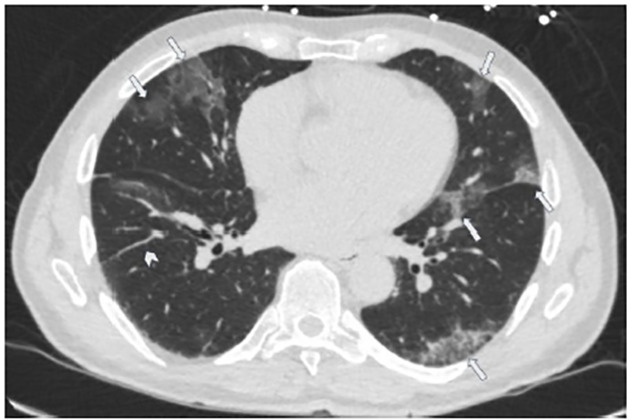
Chest computed tomography (axial image) demonstrating multifocal peripheral bilateral ground glass opacities (arrows) with interlobular septal thickening (arrowhead) and mild peribronchial thickening, suggestive of infectious/inflammatory airway disease which can be seen in the setting of COVID-19.
